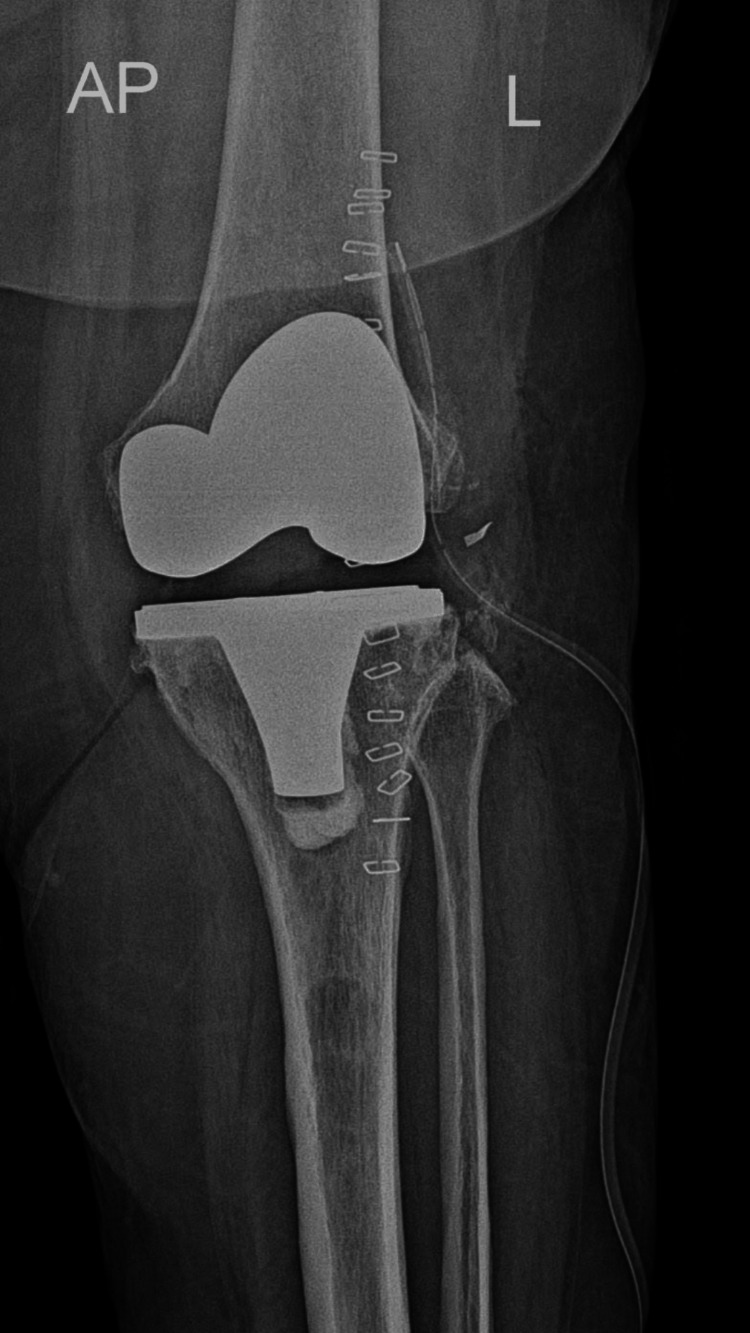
Immediate postoperative anteroposterior radiograph of the left knee Immediate postoperative anteroposterior radiograph of the left knee demonstrating appropriate alignment and positioning of the prosthetic components.
